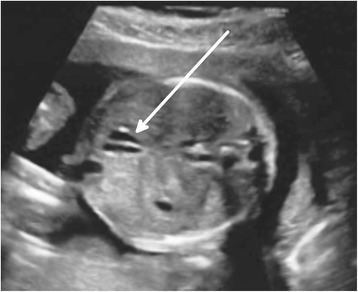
Prenatal ultrasound on the 21st week of gestational age: Transverse axial image of the fetal abdomen showing 2 adjacent fluid filled structures in the gallbladder fossa. This finding is consistent with the presence of a duplicated gallbladder
